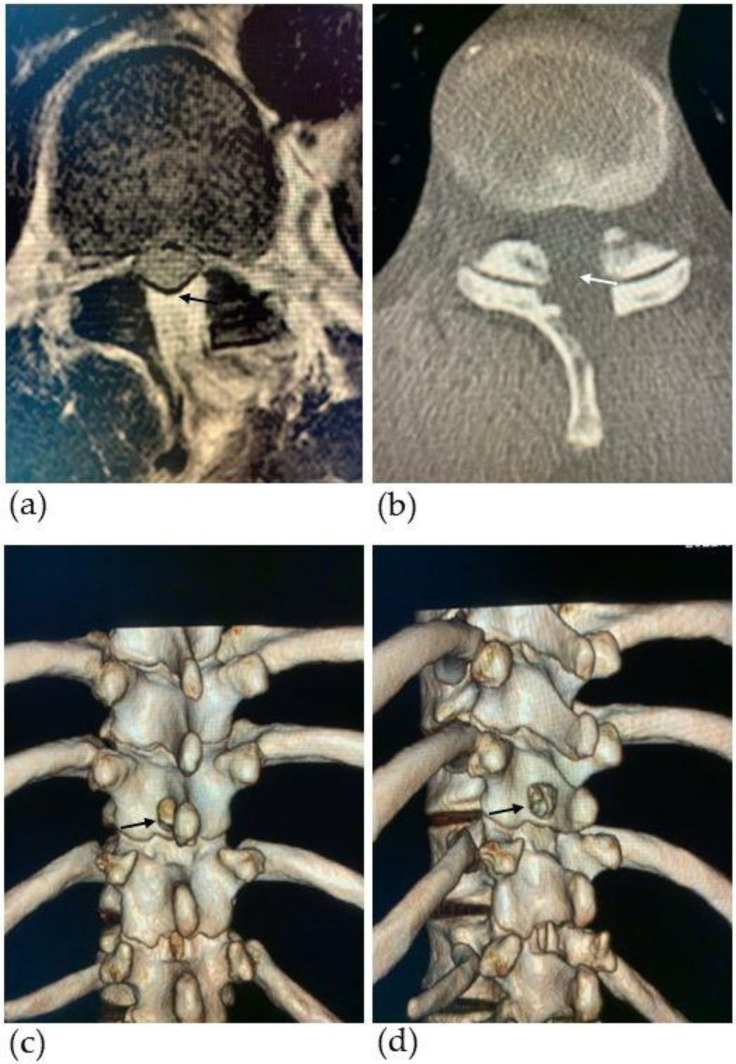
Postoperative magnetic resonance images (MRI) and computed tomography (CT) images. (a) Axial T2-weighted MRI of the thoracic spine at the T10-11 level shows spinal cord decompression (black arrow) with resection of ossification of the ligamentum flavum (OLF); (b) axial CT images of the thoracic spine at the T10-11 level shows resection of OLF (white arrow); the dorsal (c) and posterolateral (d) three-dimensional (3D) reconstruction CT images show a micro-window (black arrows) approximately 12 mm in diameter at the T10 lamina.
